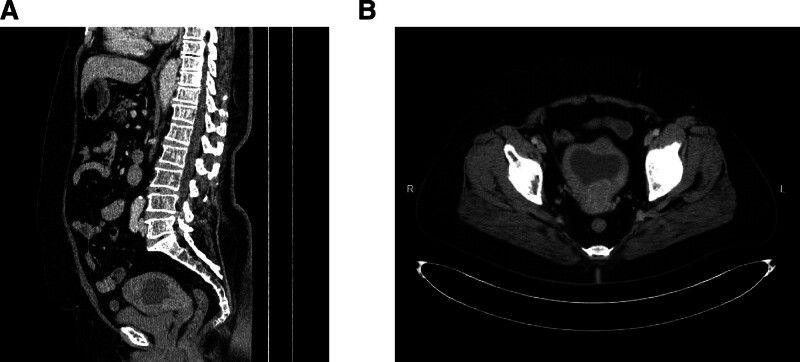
Preoperative contrast-enhanced CT of the entire abdomen reveals an irregular mass in the cervical canal, with indistinct borders and relatively uniform enhancement: (A) sagittal, (B) axial.
